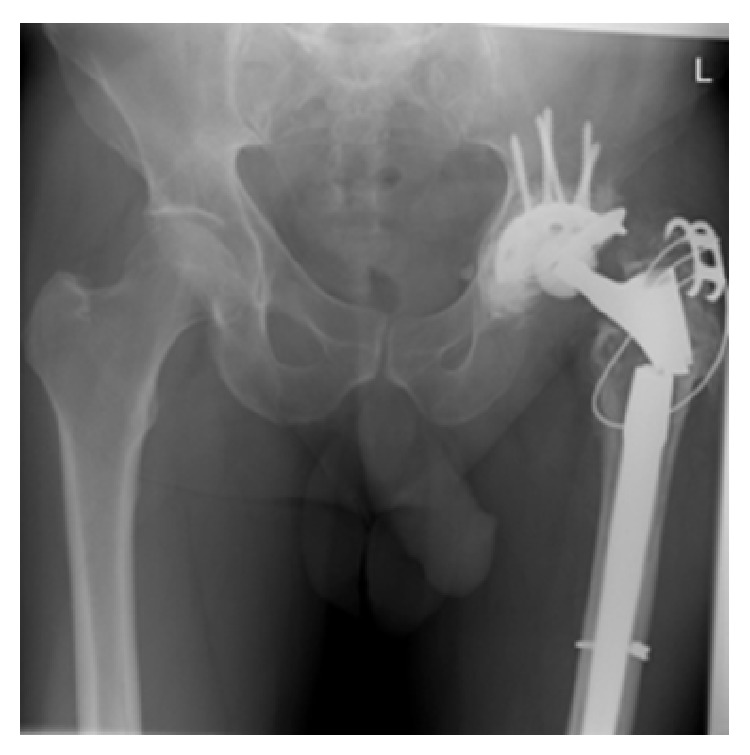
Plain AP pelvic radiograph of broken Revitan stem.
